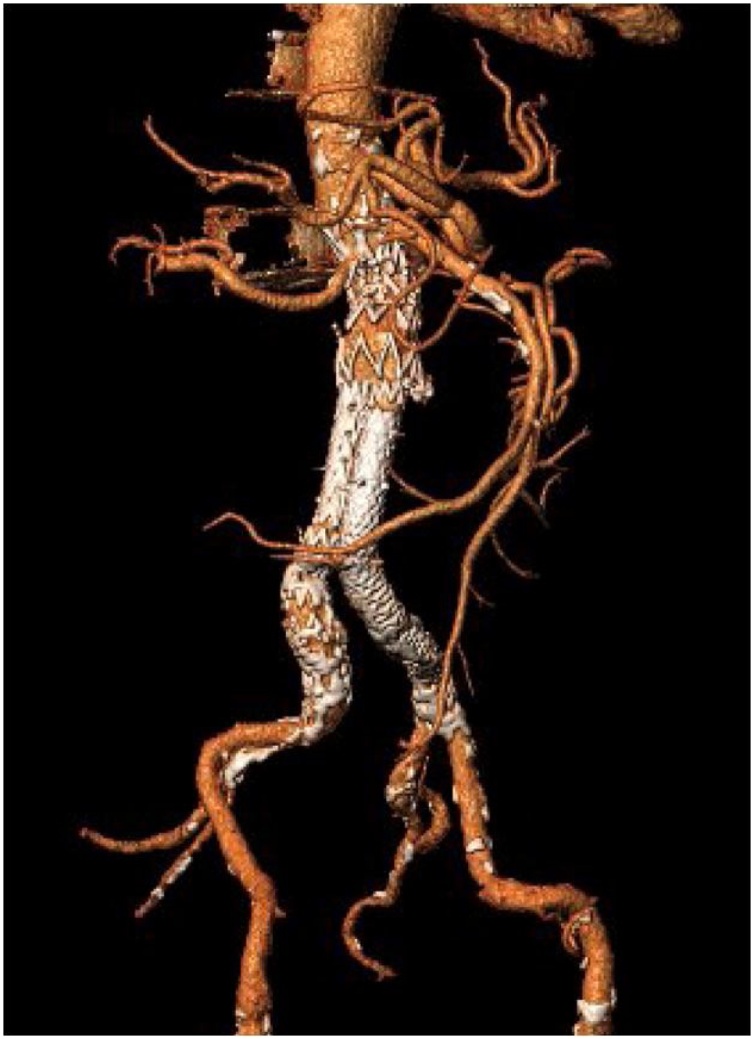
Post EVAR3D reconstruction of the previous follow up CTA.
